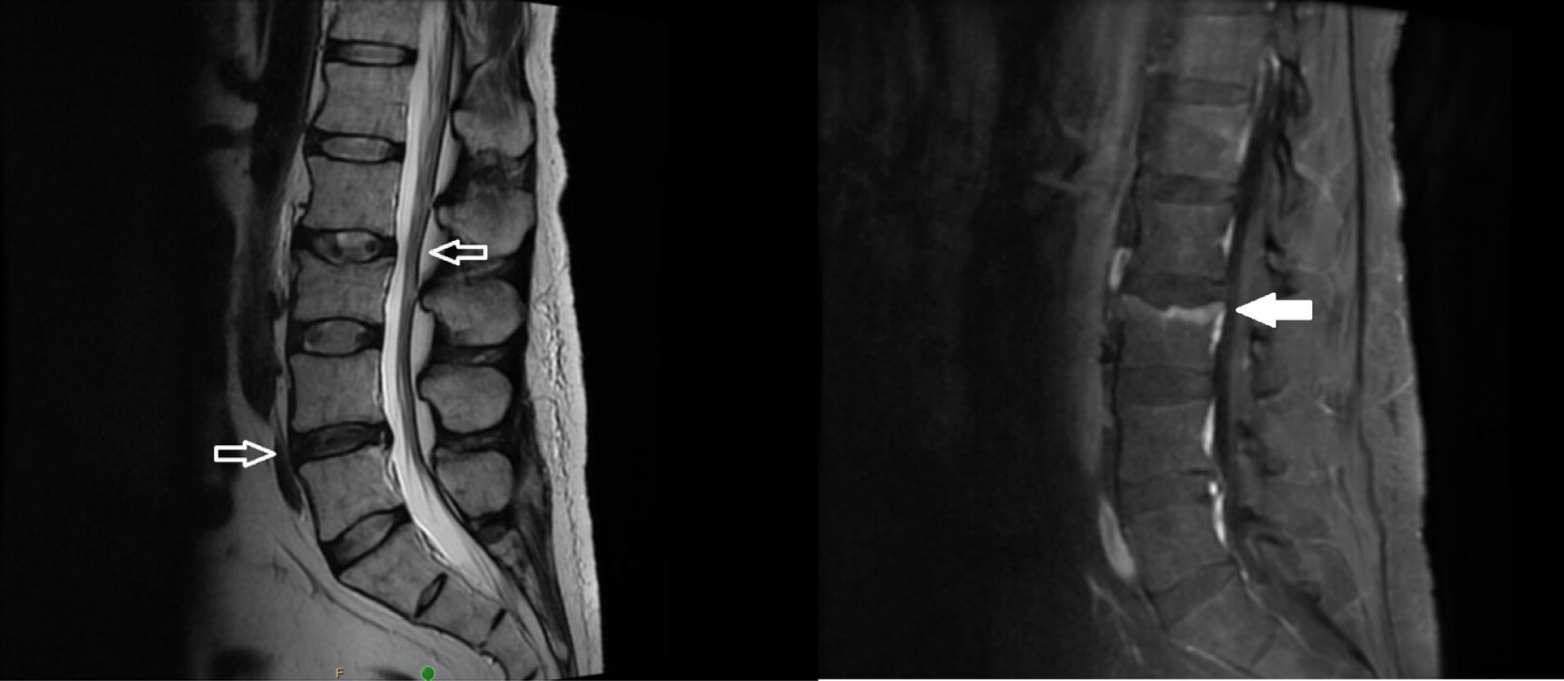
Key images from the MRI of the lumbar spine, with focus placed on the anterior disc protrusion at L4-L5 and the posterior disc protrusion at L2-L3 (nonshaded arrows), as well as the chronic end-plate fracture at L3 (shaded arrow).
